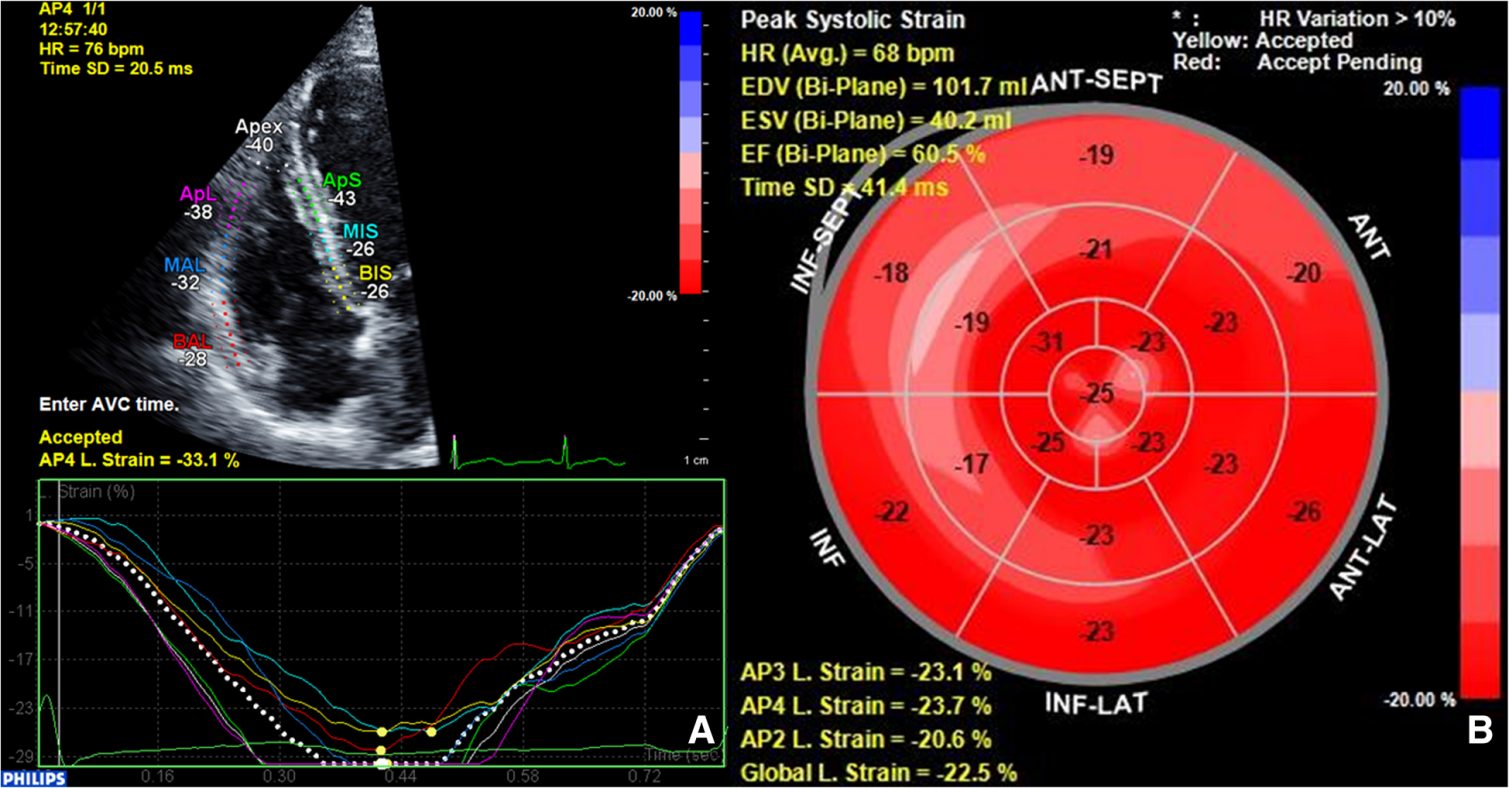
Speckle tracking and strain measurements performed on transthoracic echocardiogram performed approximately 1 h after carfilzomib administration on the 1st day of 6th cycle of treatment. Panel (a): Speckle tracking measurements performed of the right ventricle with a longitudinal strain rate of − 33.1% (normal for age, gender and software). Panel (b): Polar map of left ventricular strain measurements of transthoracic echocardiography Speckle tracking measurements estimated a biplanar LV ejection fraction of 61% with normal strain values with a global longitudinal strain of − 22.5% (normal for age, gender and software)
